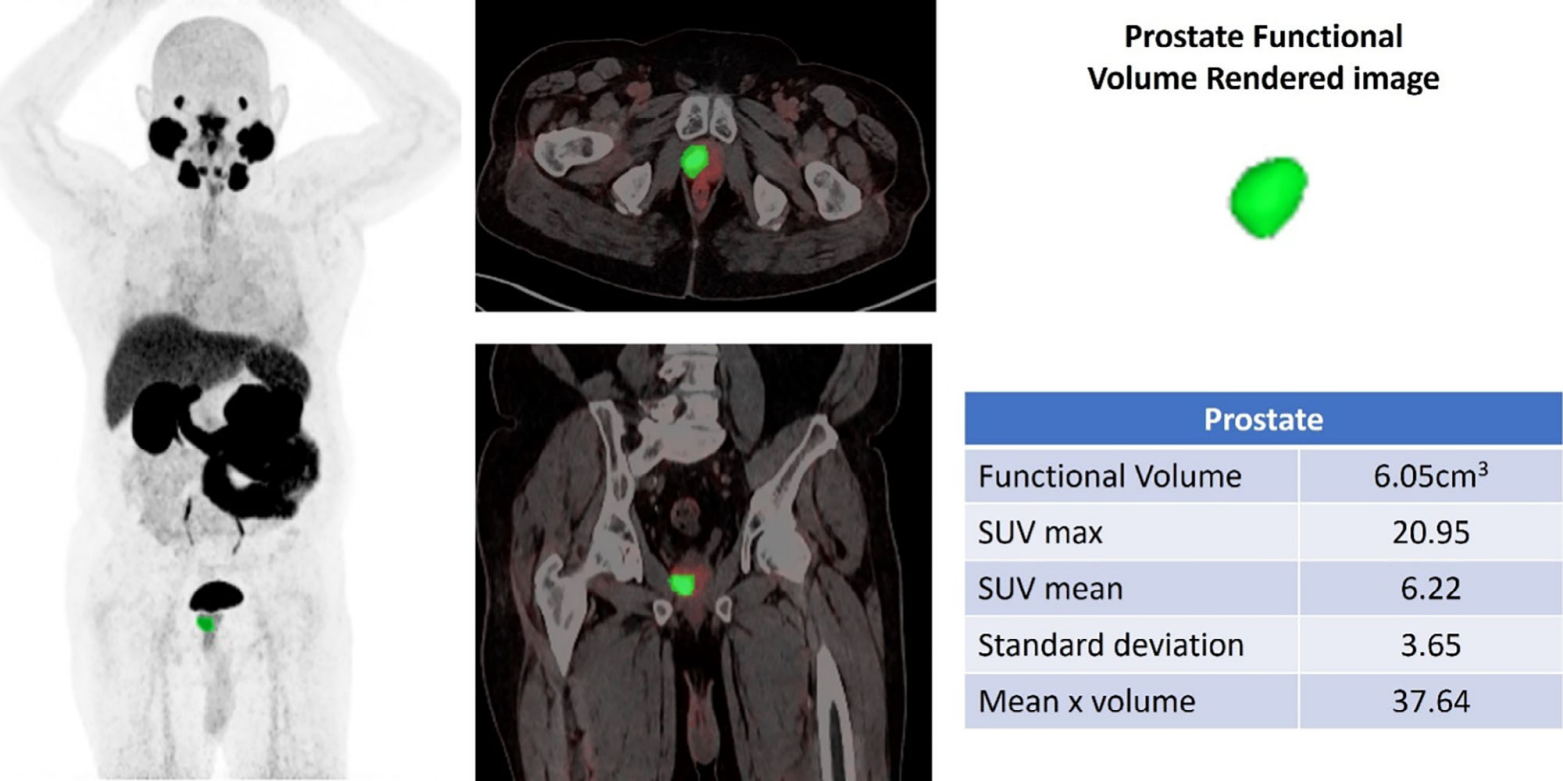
^68^Ga‐PSMA PET showing localized prostate lesion with functional volume of 6.05 cm^3^ with no evidence of distant metastasis.
